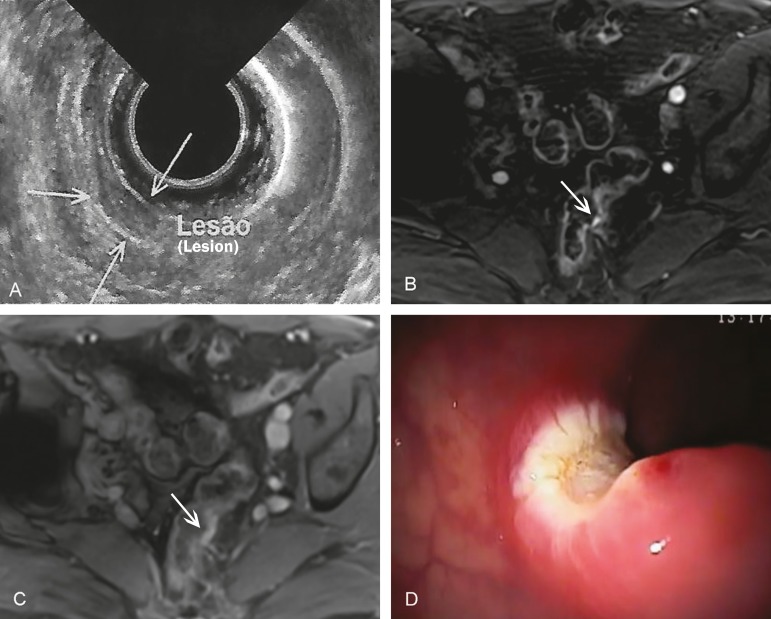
A: Endoscopic ultrasound showing a localized lesion restricted
